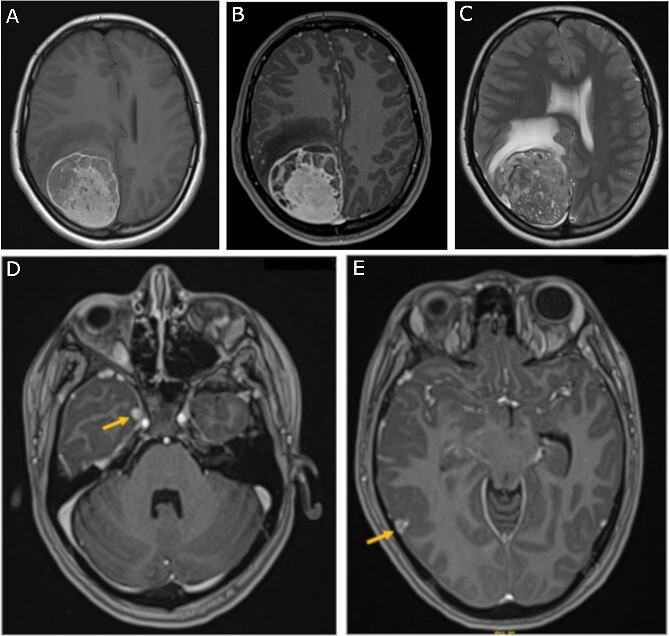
Preoperative MRI; (A) pre-contrast, (B) post-contrast T1-weighted, and (C) T2-weighted axial MRI scans show a large right parietooccipital extra-axial mass with diffusely increased T1 signal without corresponding enhancement; (D, E) axial post-contrast T1-weighted MRI scans show two discrete lobular lesions along the medial aspect of the right temporal lobe and posterior cortical surface of the temporal lobe respectfully.
